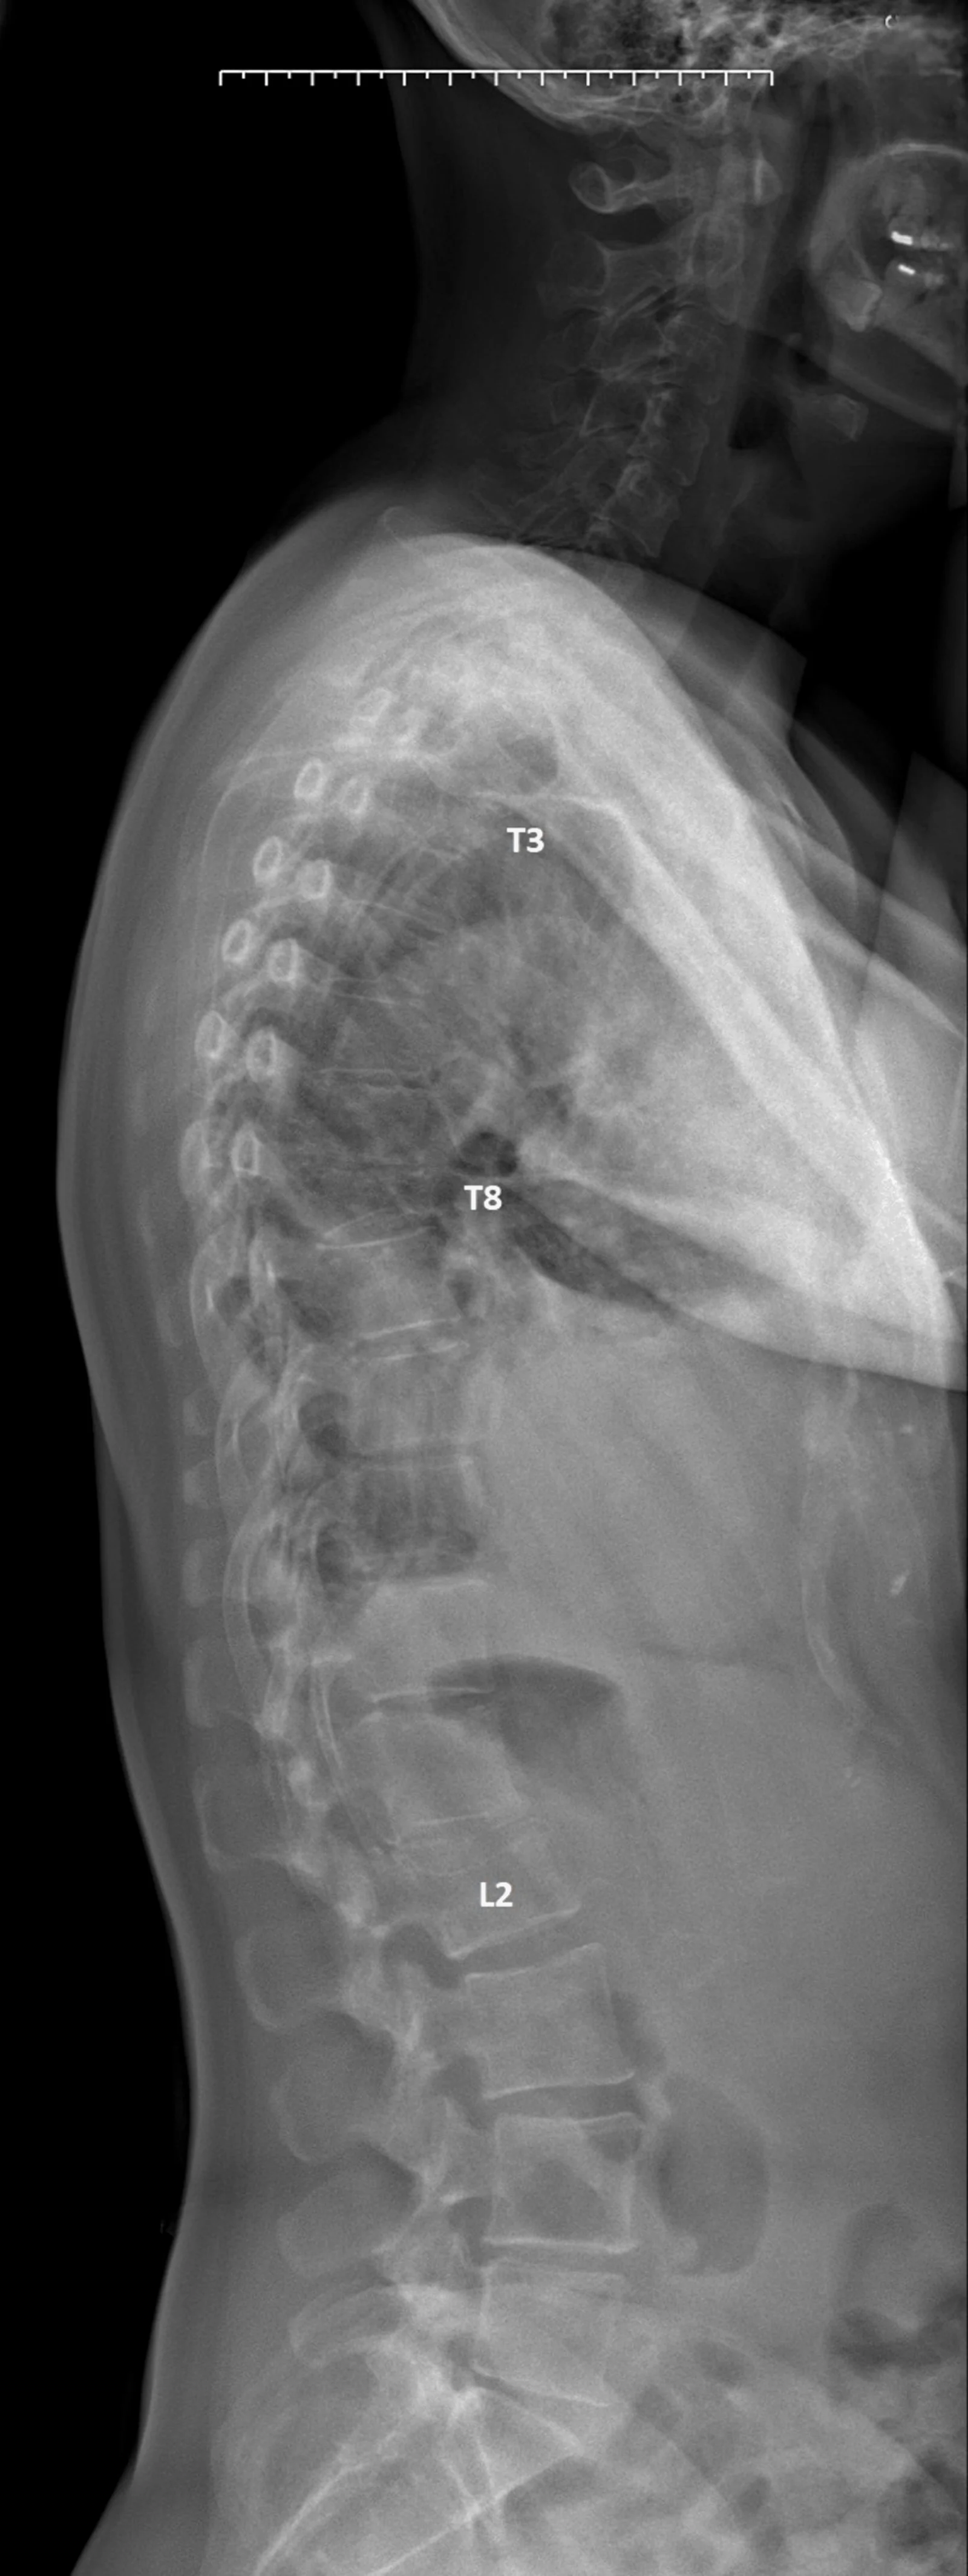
Sagittal thoracic and lumbar plain radiographs demonstrating multi-level structural compromise Significant loss of anterior and posterior vertebral body height is noted at the T3, T8, and L2 levels, representing pathological compression fractures secondary to osteolytic metastatic infiltration.
